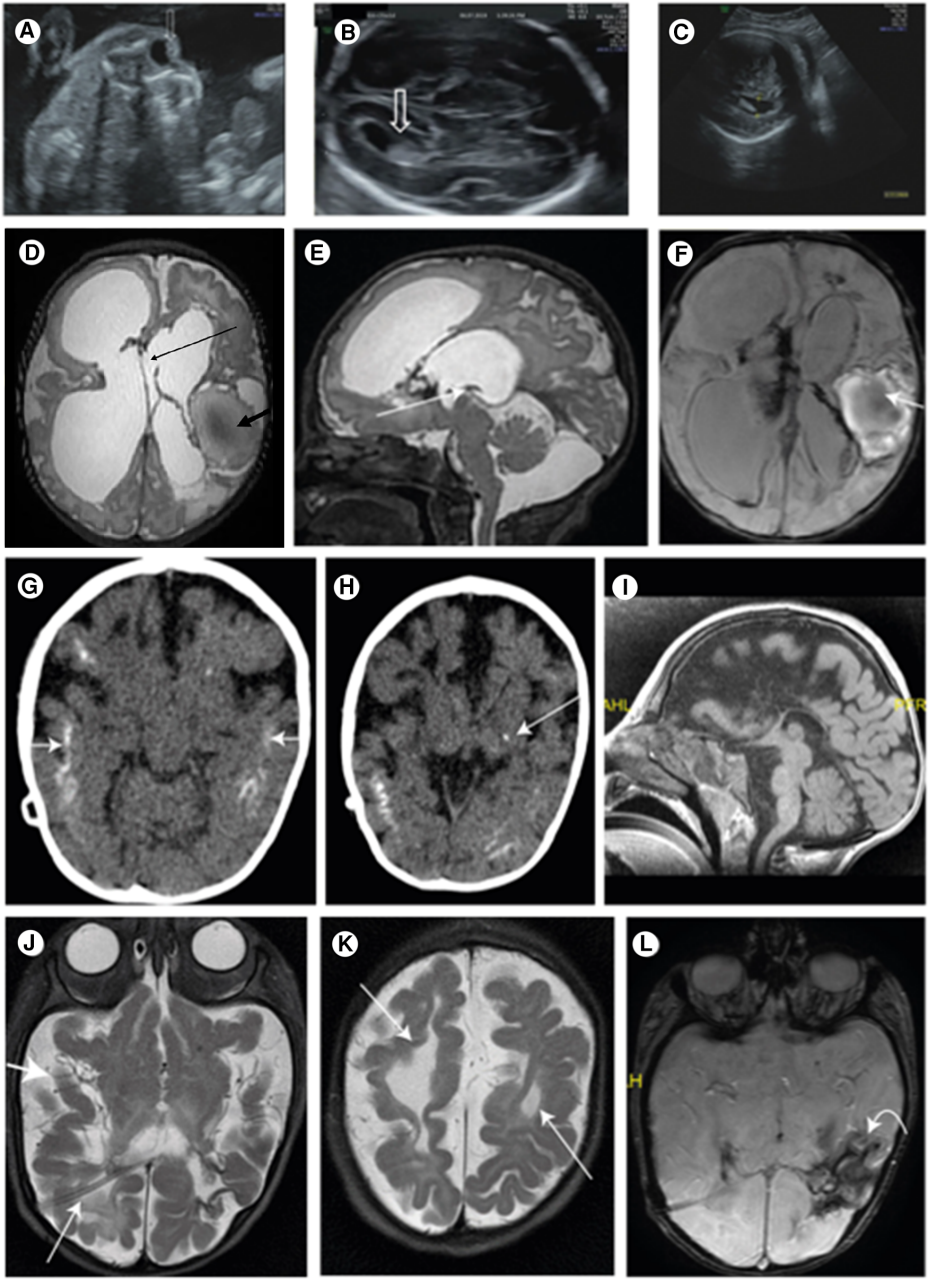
(A) Central echogenicity of the lens (arrow) indicating congenital cataract at 23 WG; (B) normal lateral ventricle (arrow) at 23 WG; (C) ventriculomegaly of 13.4 mm at 34 WG indicating the progressive nature of the disease; (D) T2 FSE axial brain. Large ventricles: Right frontal porencephalic cysts bold arrows: hemorrhagic cystic malacia. Interhemispheric cyst connecting the lateral ventricles thin arrow; (E) sagittal brain T2 fast spin echo (mri sequence name) (FSE) thin arrow: closed aqueduct; (F) swan axial brain thin arrow: hemorrhagic cavity; blood product in the lateral ventricles; (G) brain CT scan without contrast media: after VP shunt. Bilateral, extensive, cortical calcifications (white arrows); (H) a spot of basal nuclei calcification on the left (white arrow); (I) MRI after VP shunt. Mid sagittal. The corpus callosum is not seen; (J) MRI after VP shunt. Shunt in the right side (RT) lateral ventricle (thin arrow). The third and lateral ventricles are very small, and the subarachnoid spaces are enlarged (thick arrow), due to decrease of the pressure; (K) the cystic malacia changes decreased in size (thin arrow); (L) intracranial blood products are more clumped (curved arrow).
